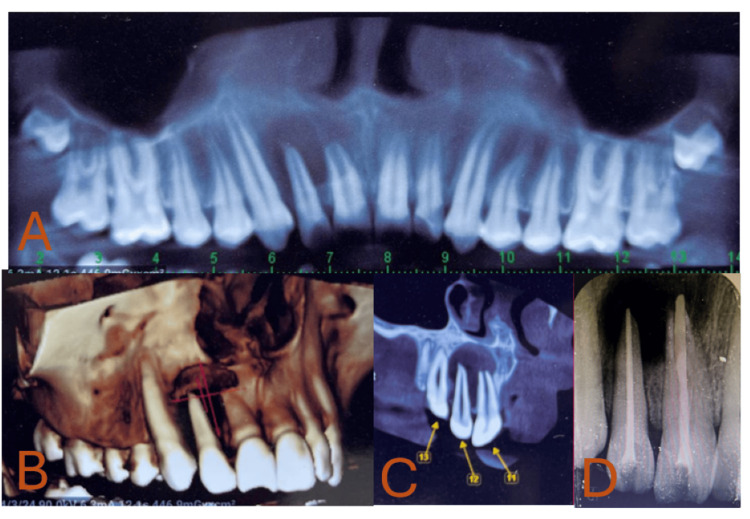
A) Unilocular radiolucency seen in CBCT; B) panoramic view; C) CBCT shows nasal floor was unbroken, and radiolucency was observed in the sagittal and coronal planes with respect to 11 and 12; D) root canal treatment done in relation to 11 and 12 CBCT: cone-beam computed tomography
